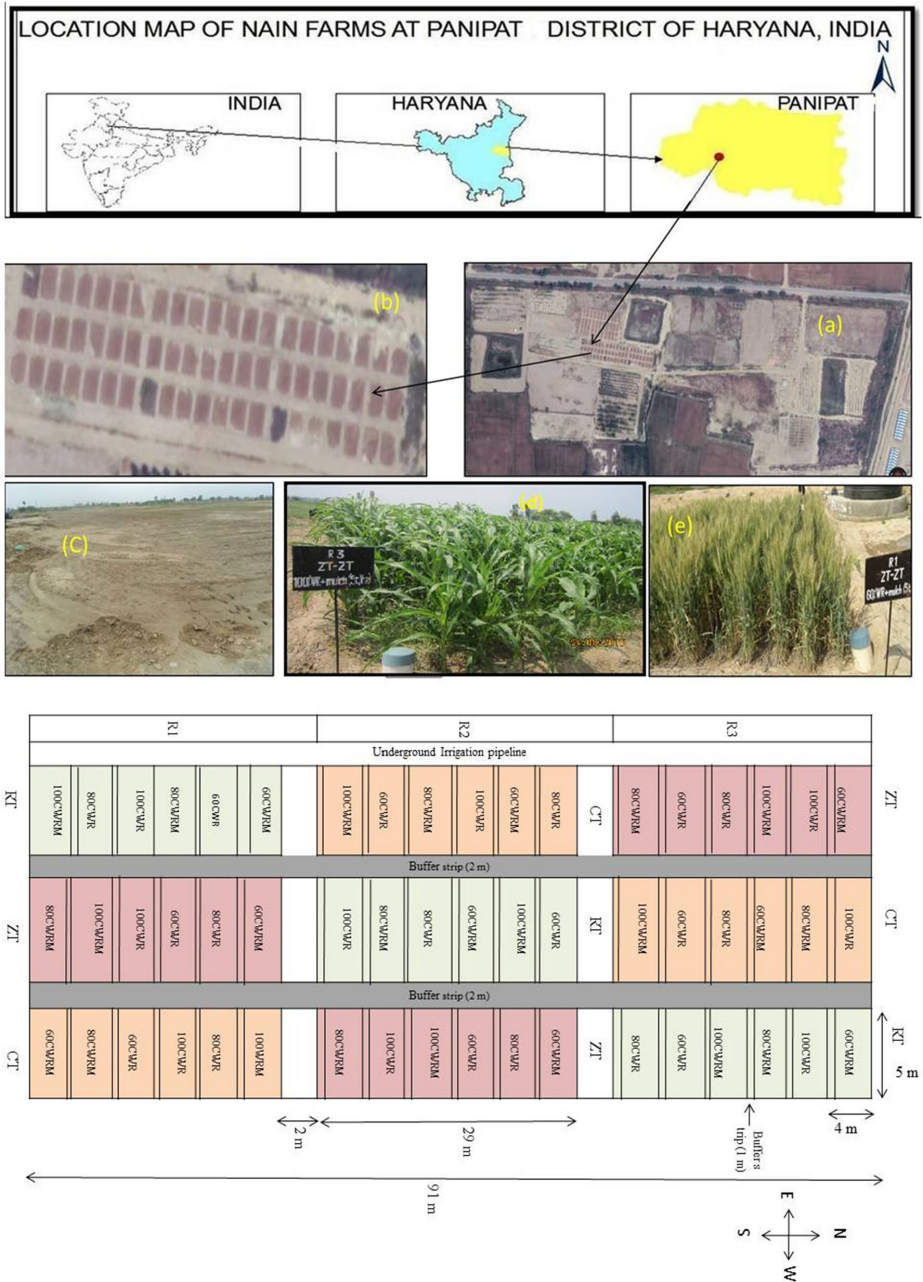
(A) Location map of field experiment at Panipat, Haryana, India; (a) CSSRI experimental Farm, Nain, Panipat, Haryana; (b) Experimental field; (c) Barren field before cultivation May 2014; (d) *Kharif* sorghum; (e) *Rabi* wheat; (B) Layout of field experiment. Main Plot: 29 m × 5 m; Sub-plot: 4 m × 5 m; between main plot 2 m buffer strip; between two sub plot-buffer strip of 1 m; ZT, zero tillage; CT, conventional tillage; RT, reduced tillage; 100, 80 and 60, per cent water requirement of wheat crop, irrigation applied only in wheat, sorghum was grown as rainfed; M, 5 Mg ha^−1^ rice straw mulch applied during wheat.
